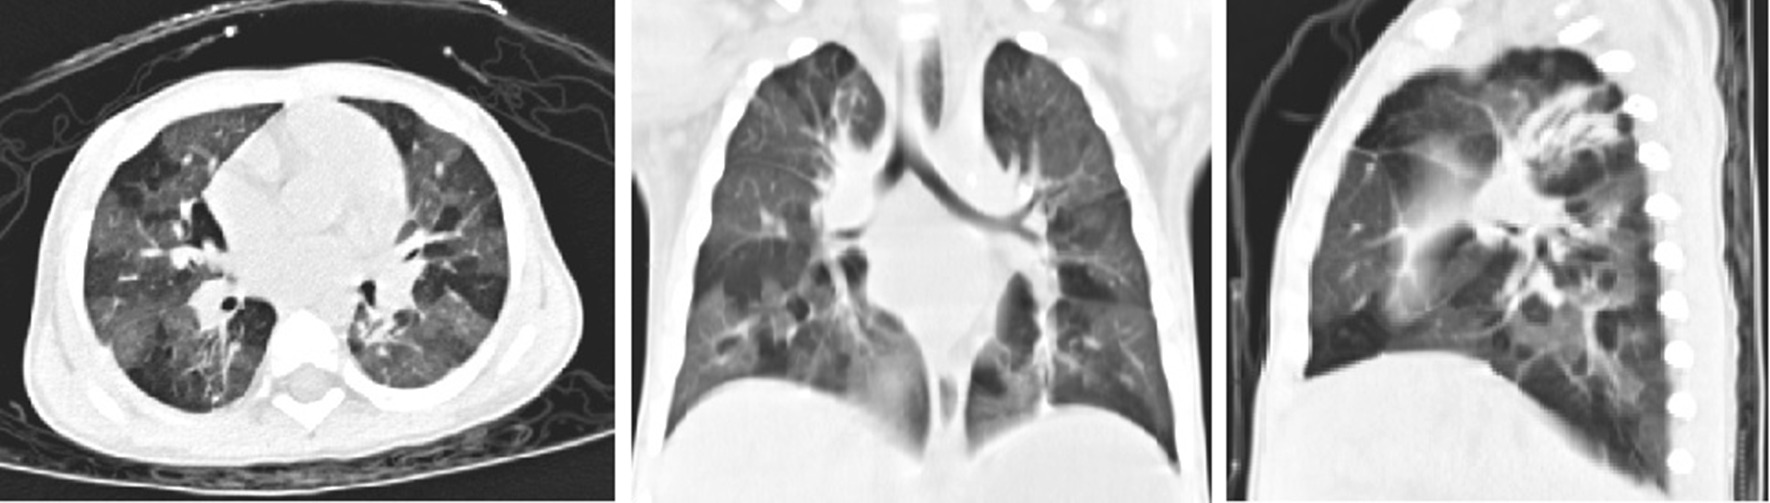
High-resolution computed tomography scan of the chest during follow-up showing bilateral mosaic ground-glass patterns with air trapping in a 22-month-old child with severe adenovirus pneumonia
